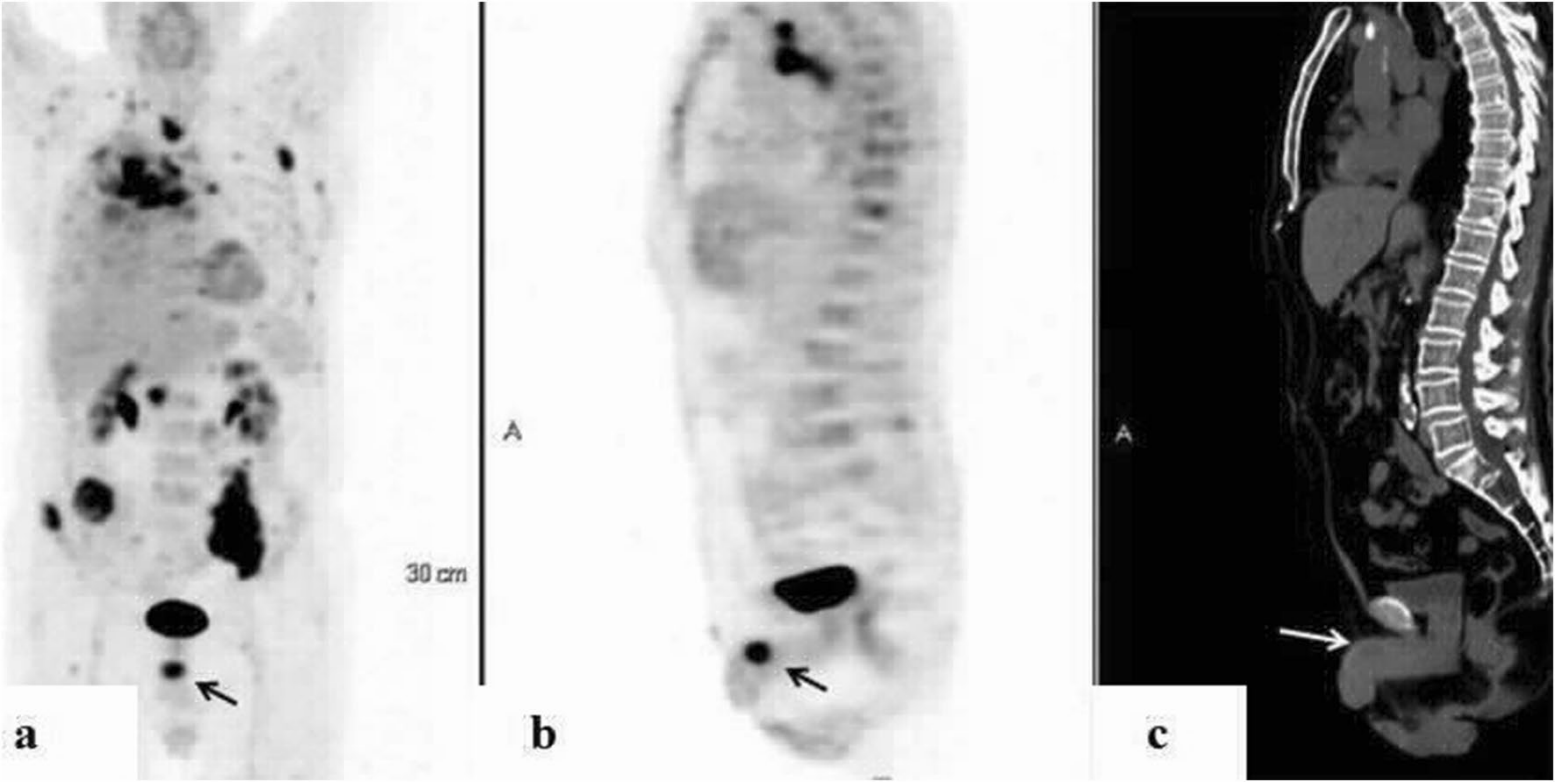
18-FDG PET/CT: maximum intensity projection (MIP) (a) representative PET (b) and CT (c) axial slices demonstrating focally increased uptake in the penis. A 79-year-old man with metastatic non-small cell lung carcinoma and a prior history of prostate cancer who had undergone prior transurethral resection of the prostate (patient #21, Table 1).
